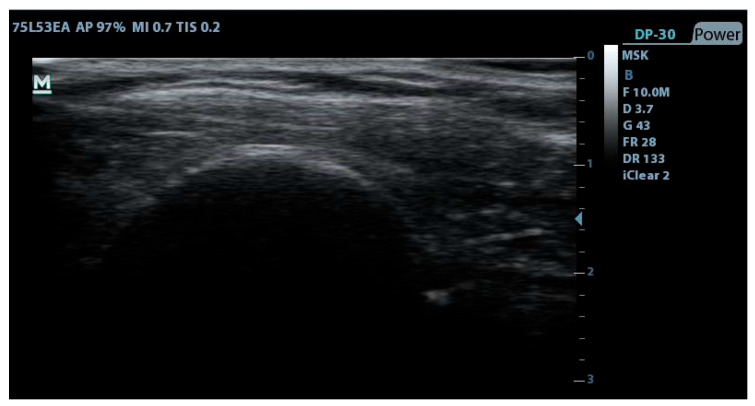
Ultrasound image of PE treatment.
